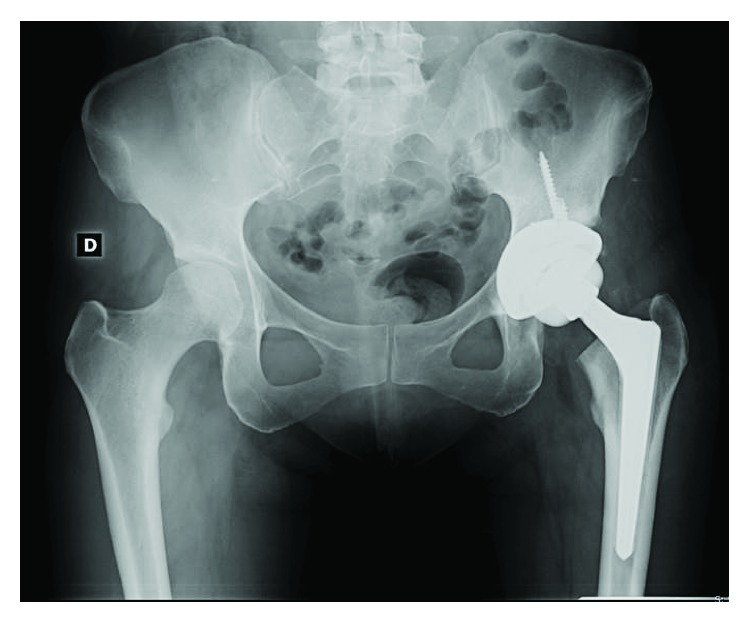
In an immediate post-RHA pelvis anteroposterior X-ray, an adequate position of the components is seen.
